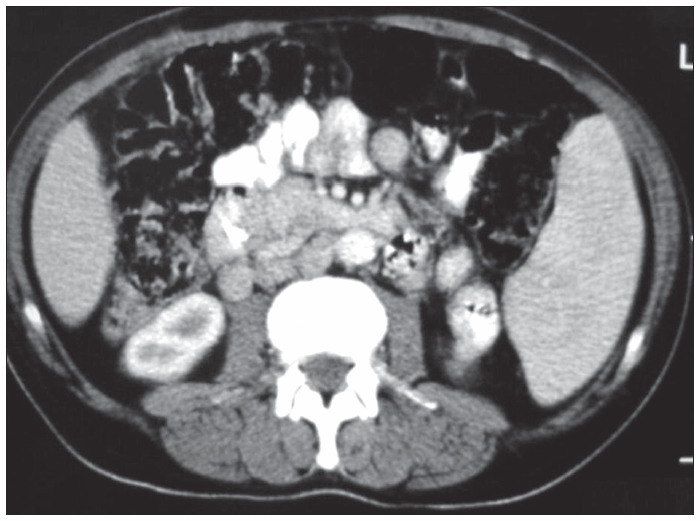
Computed tomography scan showing enlarged paraaortic lymph nodes, in a man with Hodgkin’s disease, four weeks after the placement of a plastic stent in a stricture of the bile duct.
